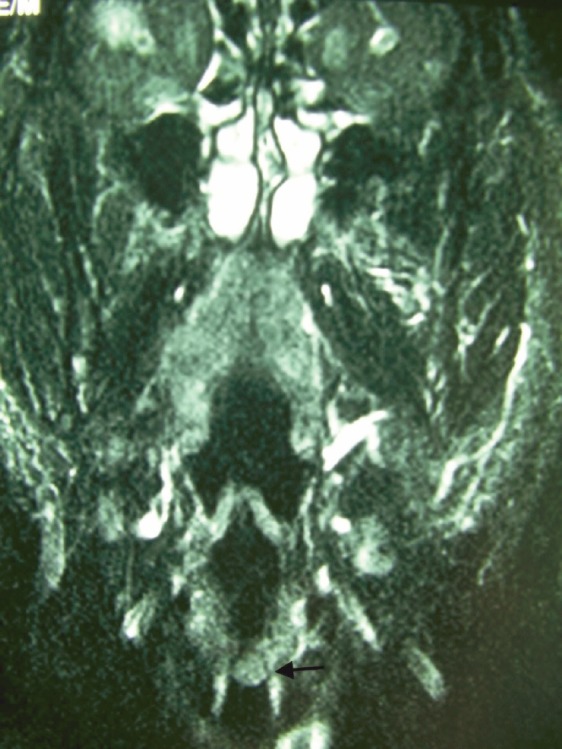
Coronal T1-weighted MRI reveals thickening of the left vocal cord (black arrow).
